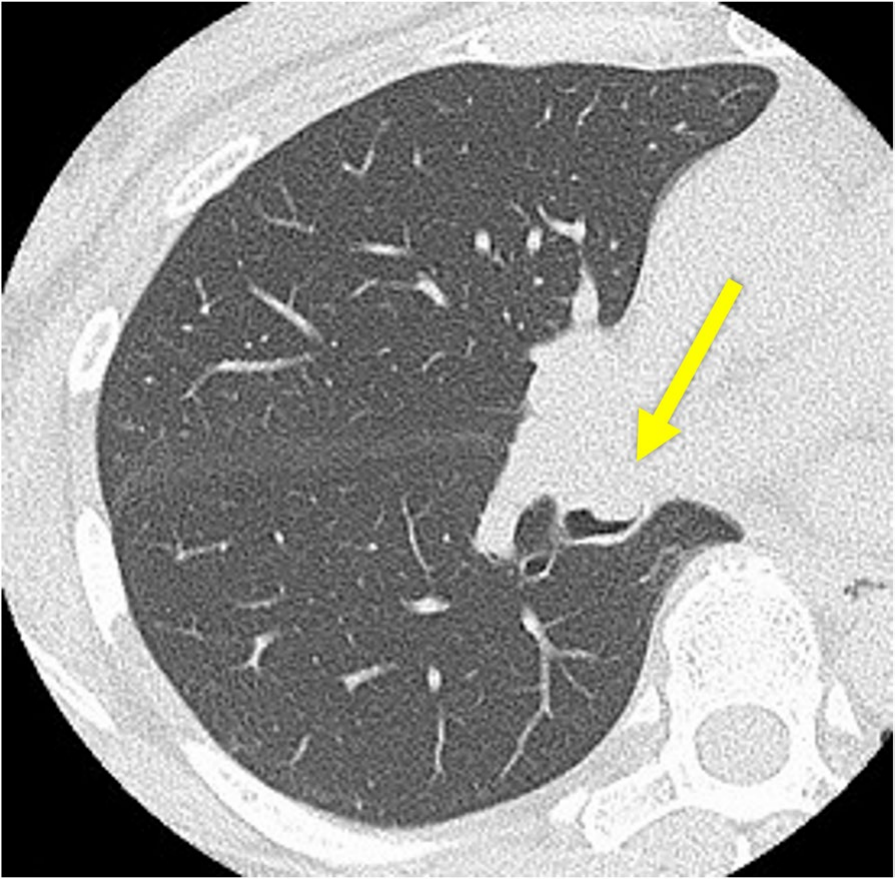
Chest computed tomography scan revealing atelectasis of the right middle lobe, and a round tumor present at the orifice of the right middle lobe bronchus that protruded into the right intermediate bronchus (arrow)
